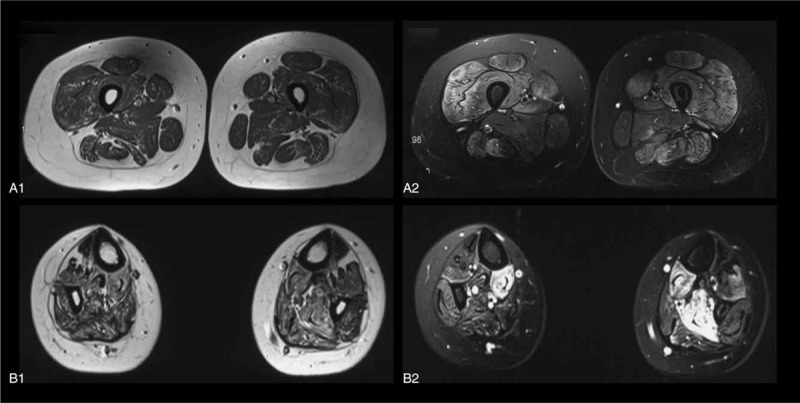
Transverse MRI images of the thighs and calves show fatty infiltration and atrophy of the muscles. The images are T1-weighted (left-hand column) and STIR sequence (right-hand column).
